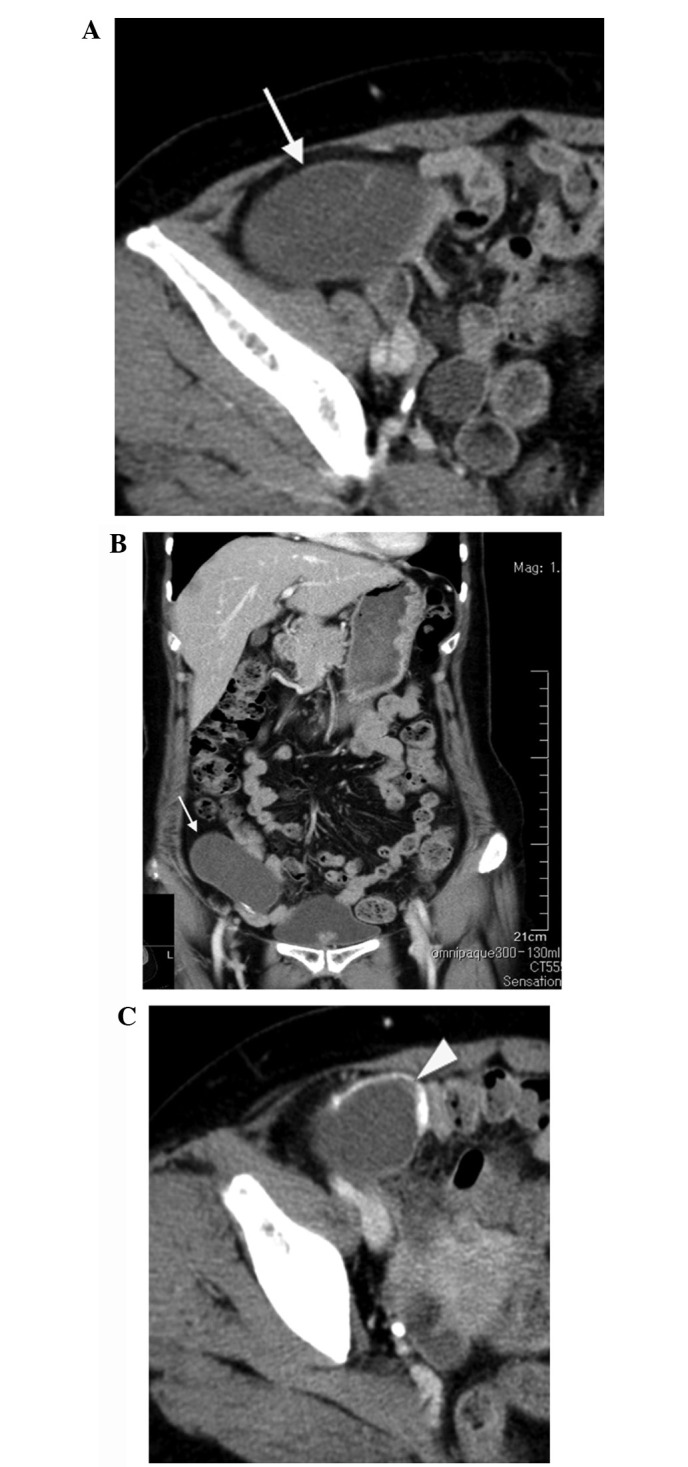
Abdominal computed tomography (CT) scans obtained in January 2011. Axial view (A) and coronal view (B) showing a 60-mm cystic mass at the site corresponding to the appendix, with no thickening or contrast enhancement in its wall. No projection was visible in the mass cavity (arrow). Another axial view (C) demonstrated calcification of the mass wall on the appendicular tip (arrowhead).
